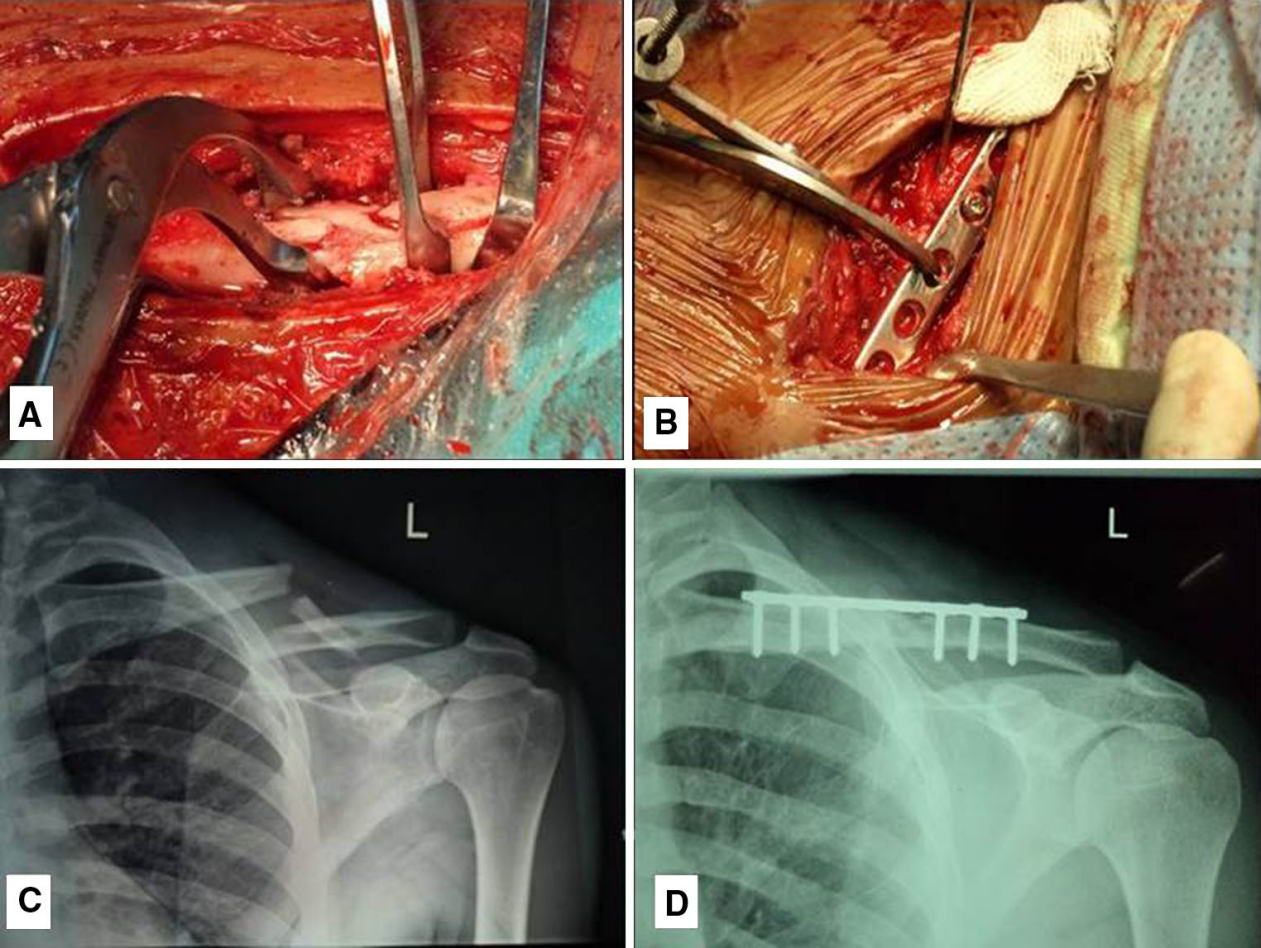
a Intra-operative fracture reduction. b Fracture fixation with 3.5 mm DCP. c Radiograph before fracture fixation. d Fracture union after 6 months of surgical treatment
